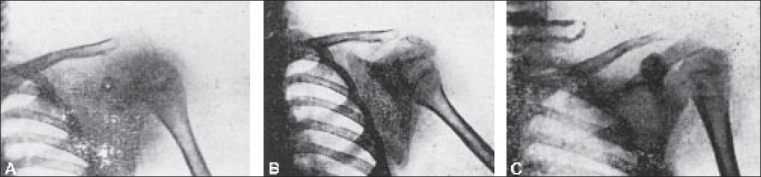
Pictures of osteosarcoma of scapula treated with X-ray therapy. (A) Before X-ray therapy, (B) After 2,000r (C) After 5,000r
